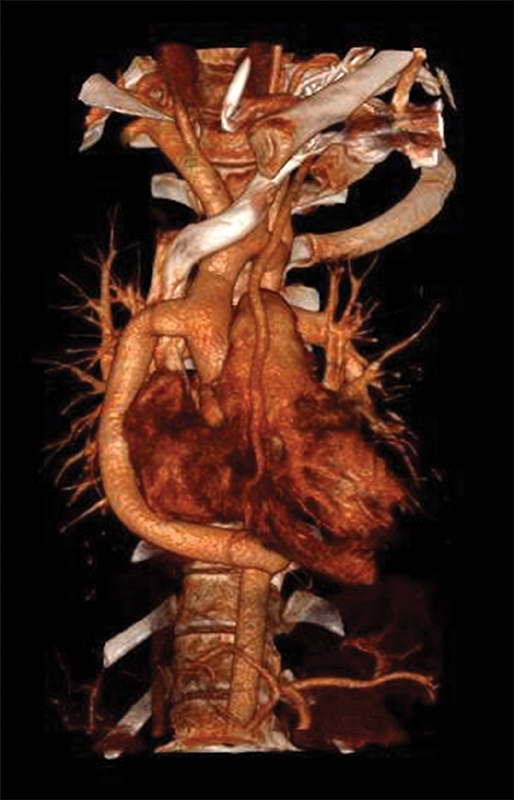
Postoperative computed tomography scan showing patent extra-anatomic conduit coursing along the right heart border.
